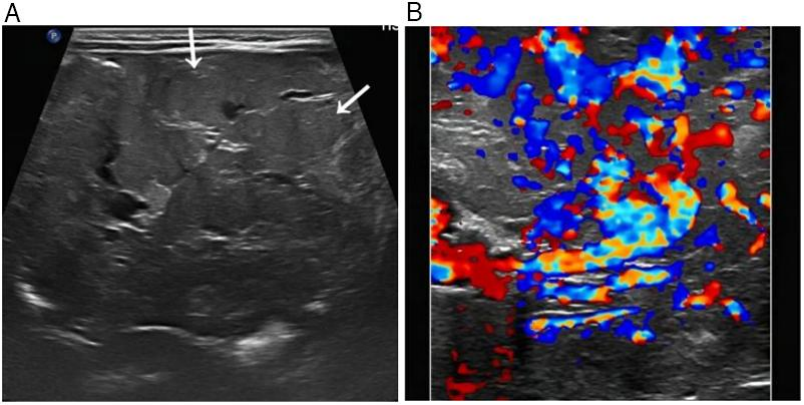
Abdominal ultrasound. (A) Grayscale image shows an extensive, ill-defined, heterogeneous mass in the mesentery(→). (B) Color Doppler image reveals rich, chaotic vascularity within the mass.
